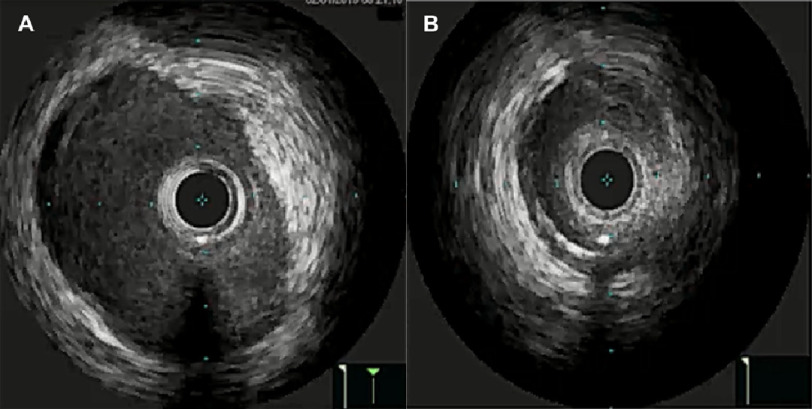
(A) - Intra-vascular ultrasound (IVUS) images obtained following intra-coronary infusion of 200mcg of nitroglycerin. Images demonstrate the distal reference area of the left main, measured at 16 mm^2^; (B) - In comparison to minimal luminal area (MLA), measured at 5.6 mm^2^.
